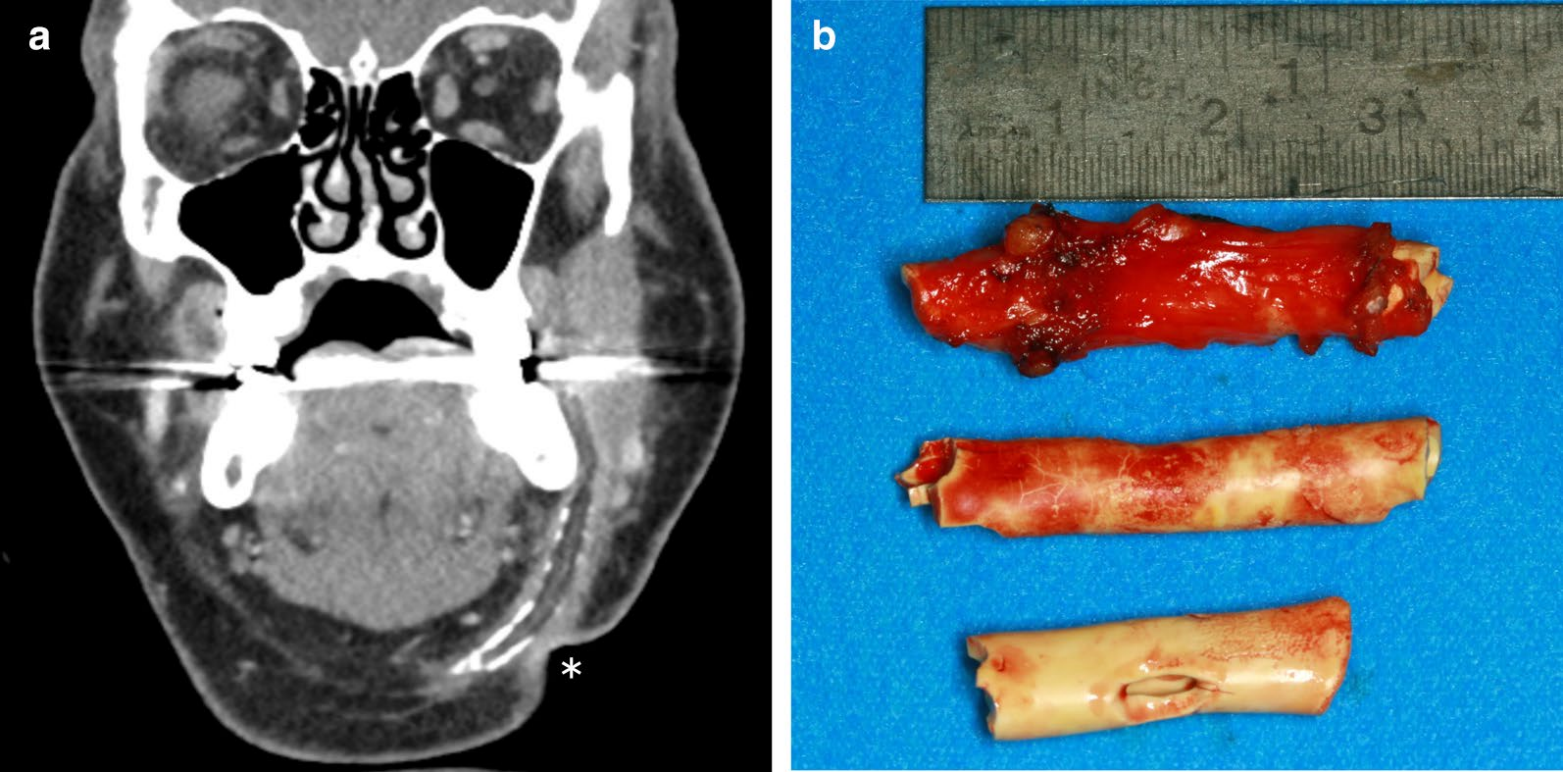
Plastic drainage tube. Coronal CT image (a) showing a tubular hypodense structure with appositional calcifications in the left submandibular space. Adjacent soft tissue reactions raise the suspicion of superinfection. Note the retraction of the soft tissue contour (asterisk), representing the old surgical scar. b Photograph of 3 parts of a drainage tube after removal
